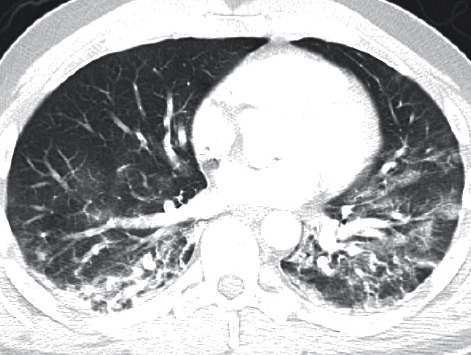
CT of the chest from 2^nd^ admission.
